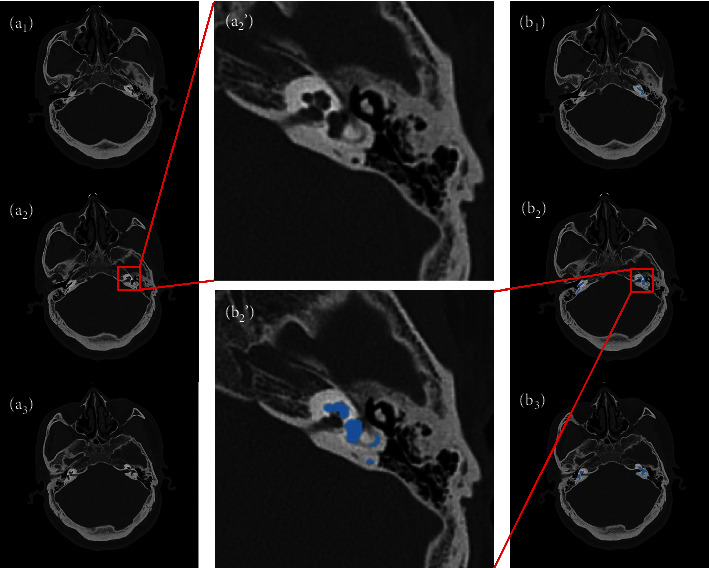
CT slices of our dataset. (a_1_), (a_2_), and (a_3_) represent the 37th, 39th, and 41st slices of a sample in our dataset. (b_1_), (b_2_), and (b_3_) are the corresponding labels; the areas where the semicircular canals are located are marked in blue. (a_2_') and (b_2_') are enlarged pictures of (a_2_) and (b_2_).
